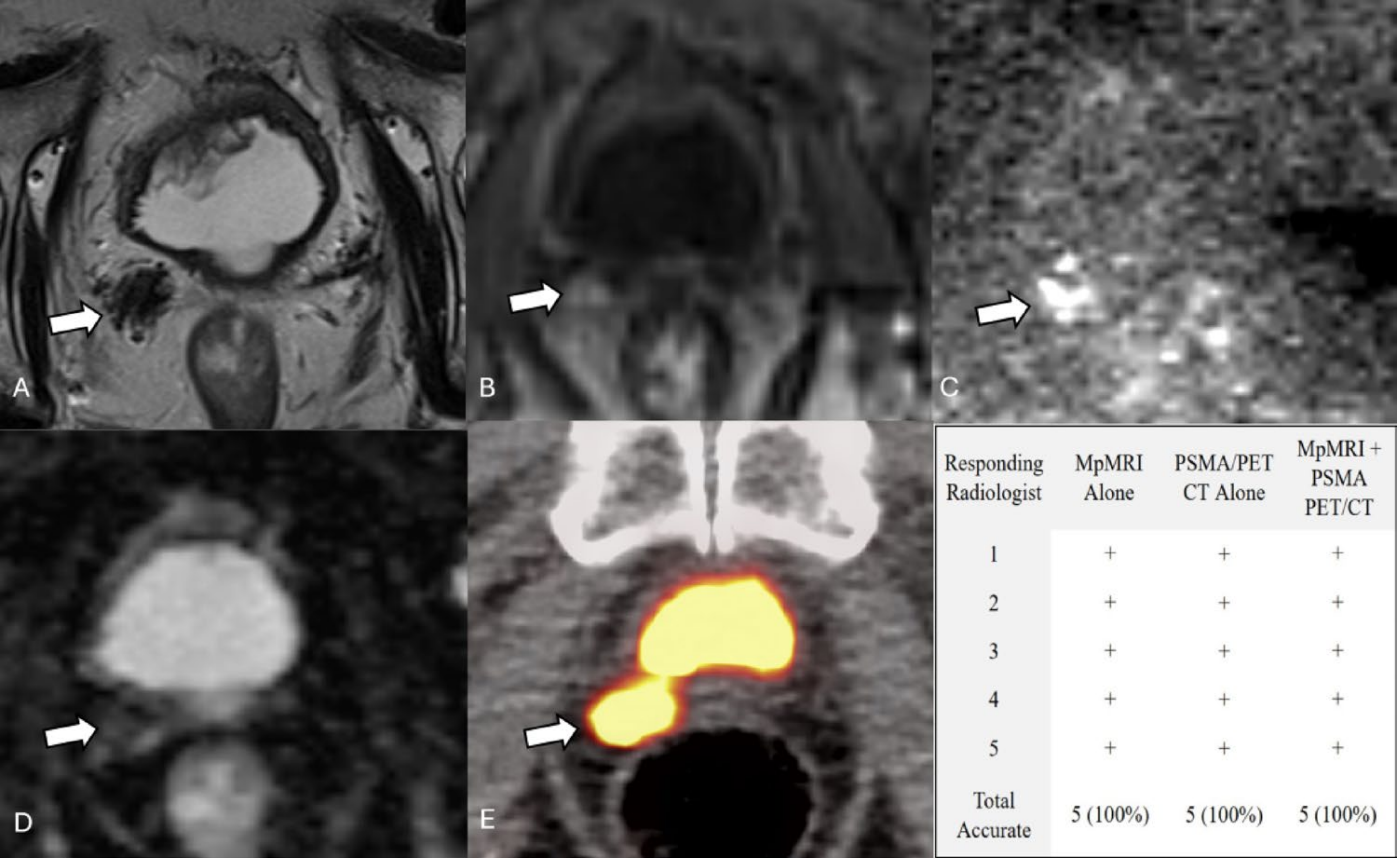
86-year-old patient with a right prostate bed lesion with focal uptake on PSMA PET/CT and focal abnormalities on mpMRI This case depicts an 86-year-old status-post prostatectomy indicated for a primary adenocarcinoma. His PSA was measured to be 0.5 ng/mL at the time of imaging. MpMRI (A T2, B Arterial enhancement T1, C DWI, D ADC) shows a > 10 mm right prostate bed focal T2 dark lesion with arterial hyperenhancement and restricted diffusion (white arrows). 18 F-piflufolastat PSMA PET/CT (E) shows focal uptake in the same region of the right prostate bed (white arrow). MpMRI and PSMA PET/CT were performed 173 days apart. In this case, performance was identical with mpMRI alone, PSMA PET/CT alone, and both modalities in conjunction (F)
